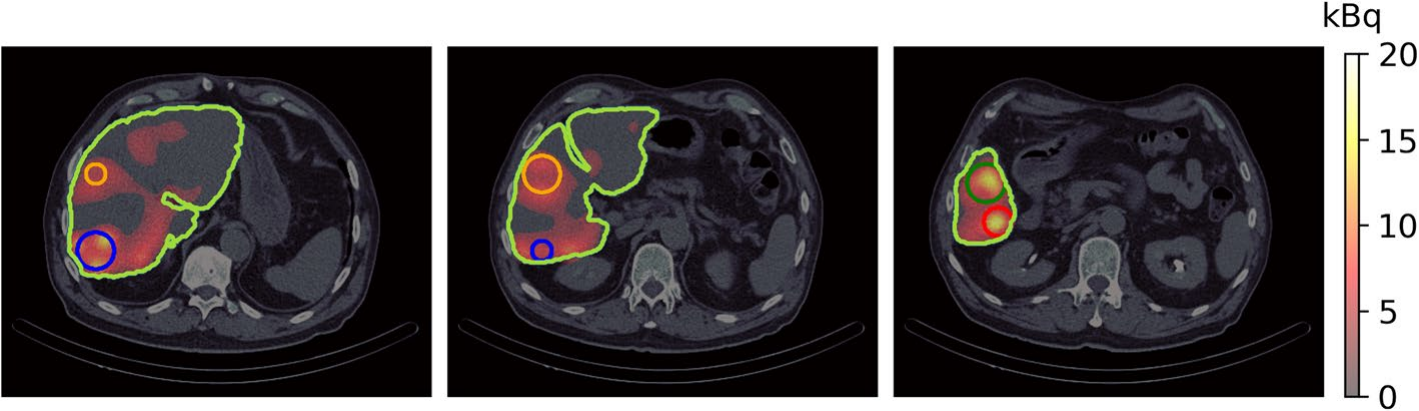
Three axial slices of fusion SPECT/CT with Liver and VOIs consider contoured
